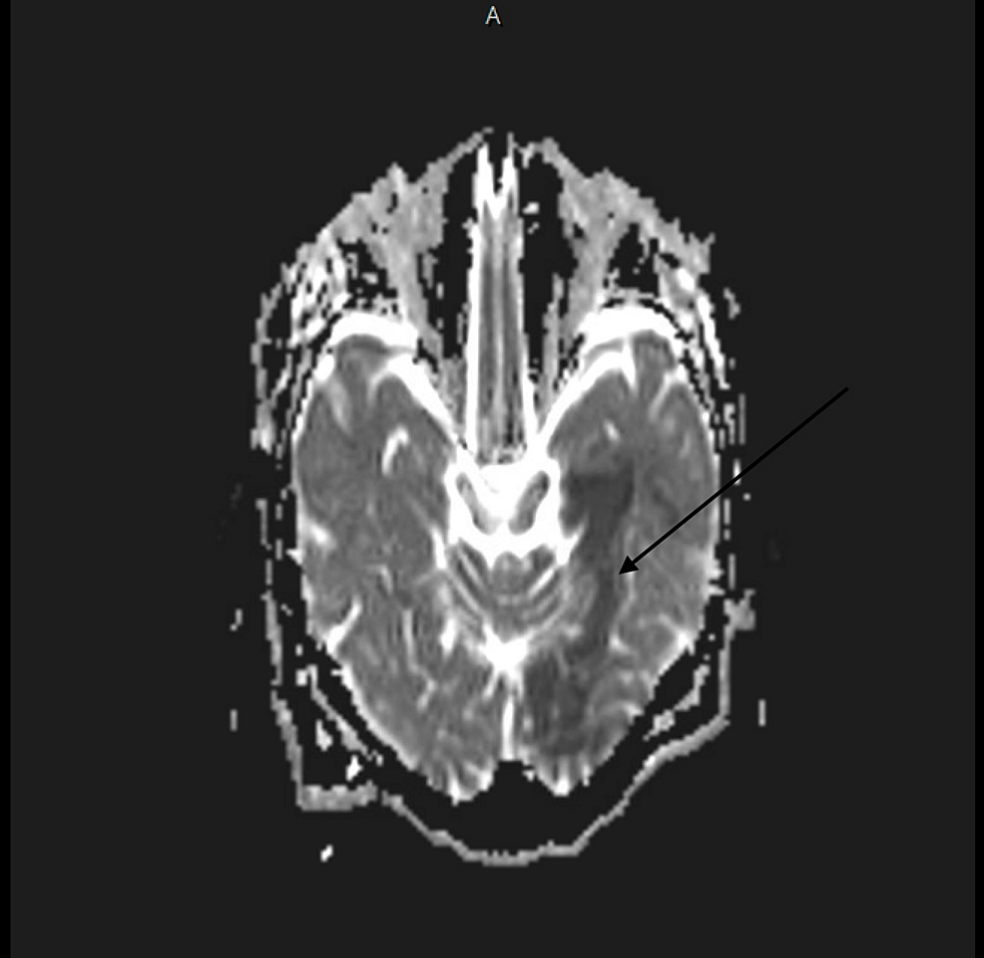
ADC MRI scan of the brain delineating the affected parts ADC, apparent diffusion coefficient
